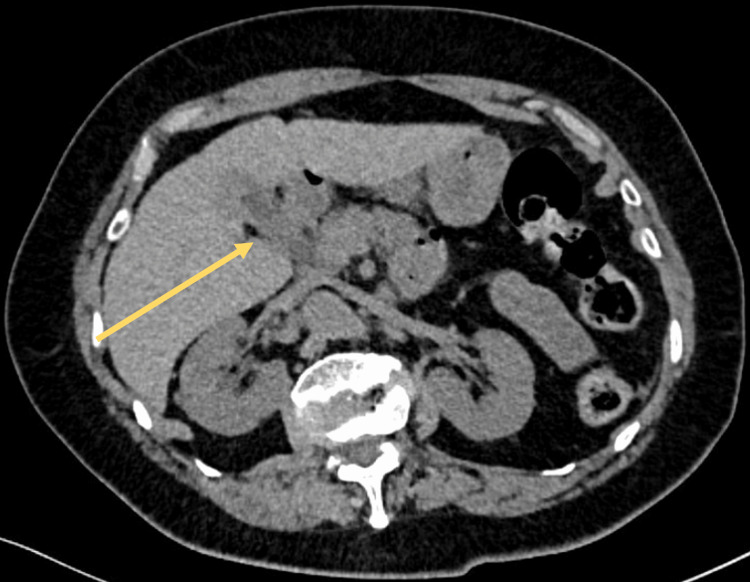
Axial View of Abdominal CT Scan The gallbladder and the common bile duct are observed to be free of air (yellow arrow), indicating the absence of air due to pneumobilia or a bilioenteric fistula. Additionally, the absence of gallbladder stones is observed. CT: computed tomography
